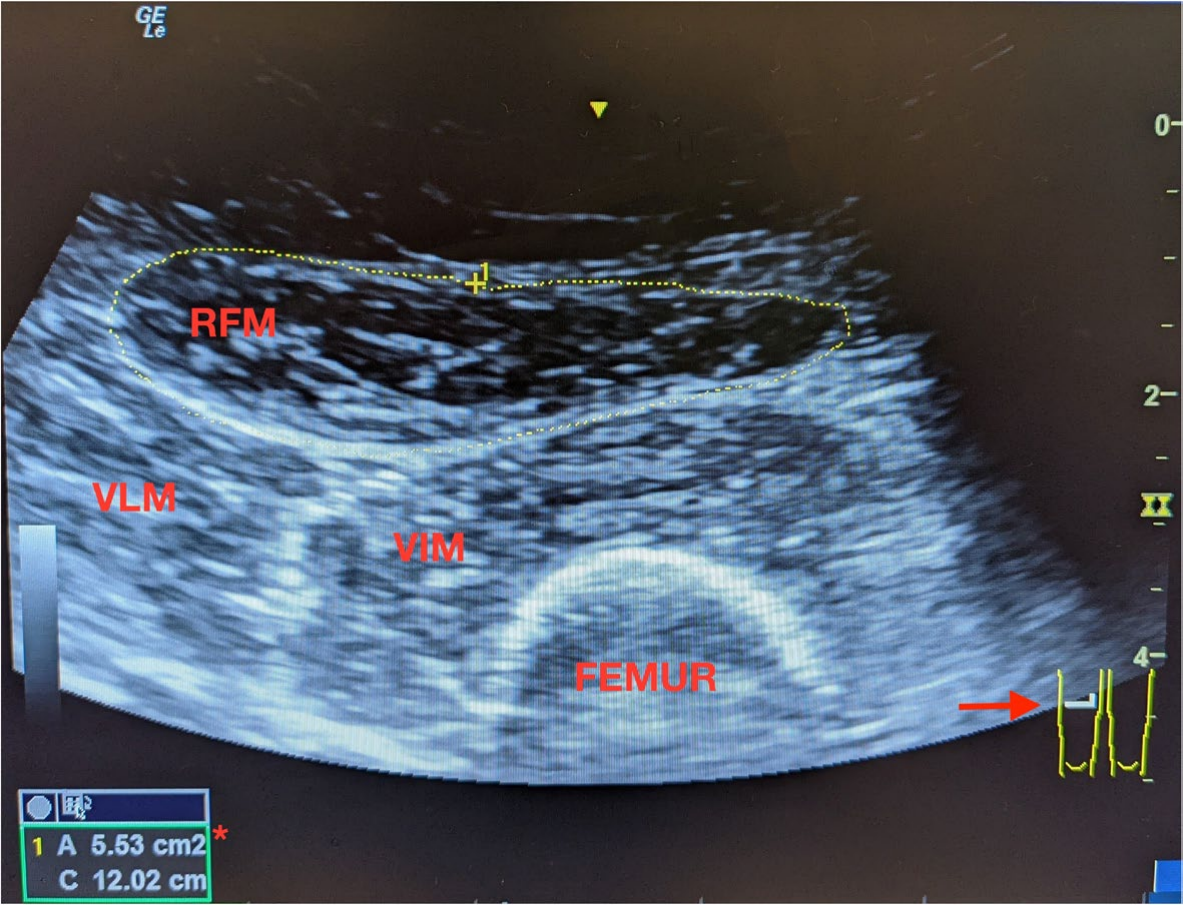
An example of the ultrasound images acquired for measurement of the rectus femoris cross-sectional area using a wide curvilinear-array transducer. RFM: rectus femoris muscle; VIM: vastus intermedius muscle; VLM: vastus lateralis muscle; Arrow: demonstrates probe location on right thigh during imaging
